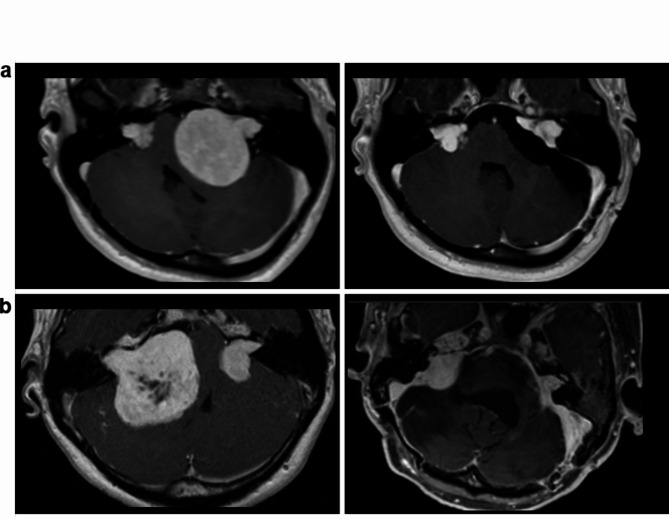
Exemplary cases of surgically induced anatomical changes and limits of the classification systems. T1-weighted contrast-enhanced axial magnet resonance images (MRI) of different NF2 patients. Two (cases a and b) other and operated NF2-associated vestibular schwannoma (VS) cases. The left column shows the preoperative, and the right column shows the postoperative images. (a) Bilateral VS, preoperative tumor size left-sided T4b/K4 and non-operated right-sided T3b/K3 VS. Surgery of the giant, left-sided VS (T4b/K4) VS was indicated and performed (regardless of hearing) due to displacement of the brainstem and fourth ventricle. A slight tumor residue had to be left to preserve the facial nerve function. The internal auditory canal (IAC) was also opened. According to Hannover, postoperative classification into T1/T2/T3a or Koos into K1/K2 is more complicated. The contralateral right side became a preoperative T4a to a “postoperative” T3a due to the relief of the brain stem. The Koos grade K2 did not change due to surgery. (b) Bilaterally operated VS, preoperative tumor size right-sided T4b/K4 and left-sided T4a/K3. The relief of the brain stem resulted in an altered configuration of the posterior fossa with almost compensatory widening of the fourth ventricle. Due to this shift in the anatomy after partial resection of the large and the opening of the internal auditory canal, it is tough to classify tumors in classification systems on both sides. This case also shows the problem of the different tilting of the MRI diagnostics.
